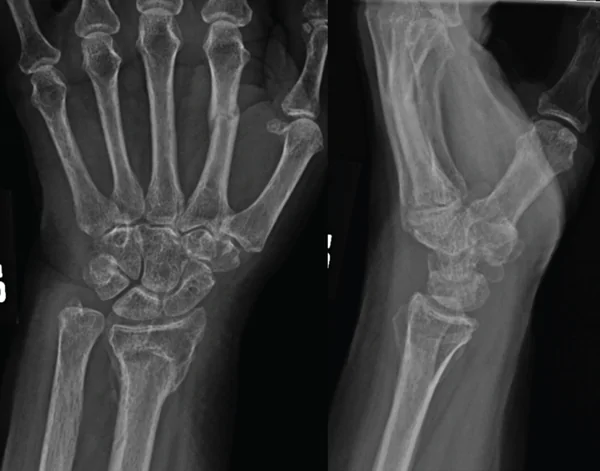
Radiographs at 6 months demonstrating healed distal radius fracture and stable union of 2nd metacarpal fracture.
